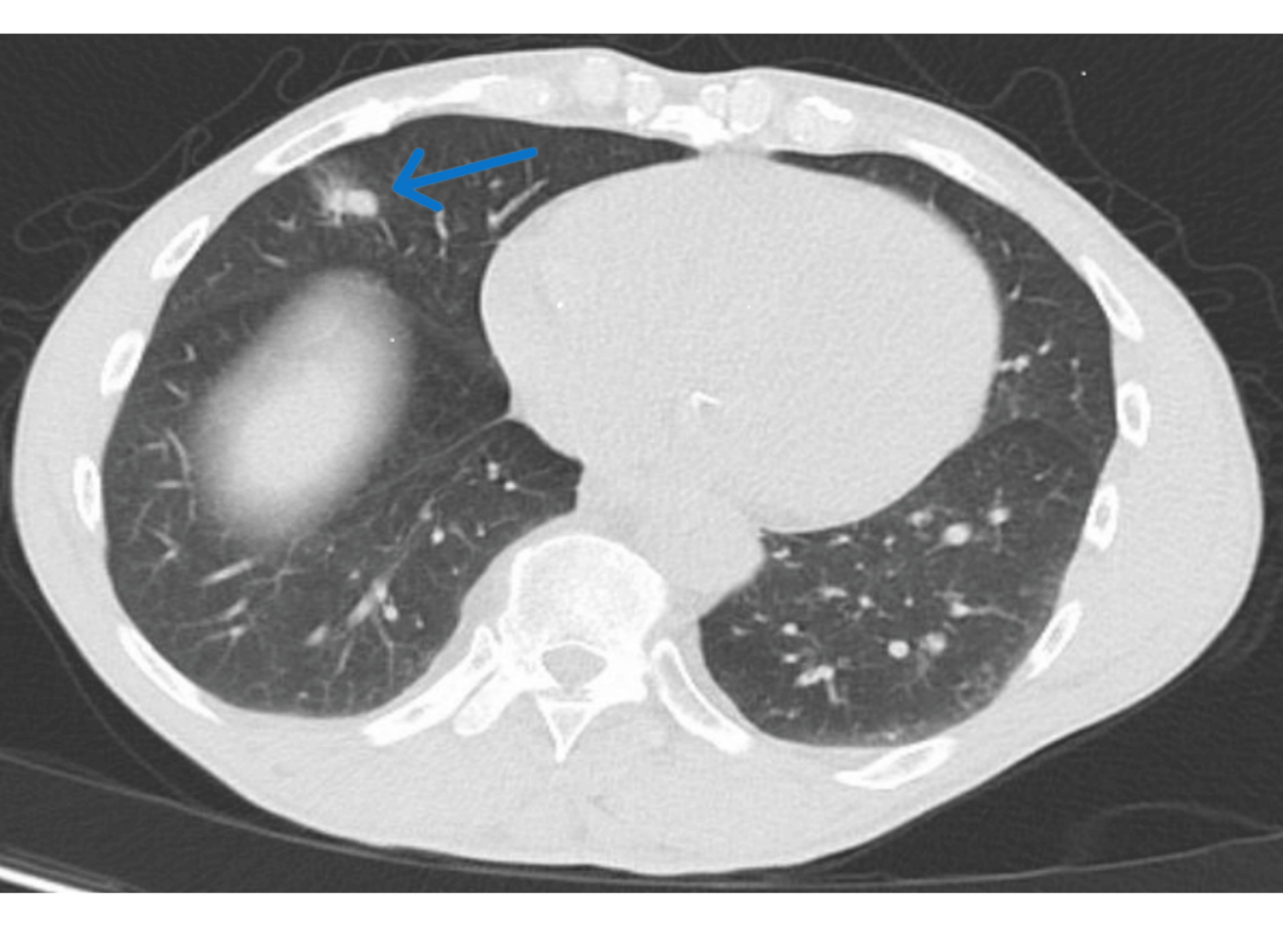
CT angiogram of chest demonstrating right middle lobe part solid pulmonary nodule (blue arrow).
